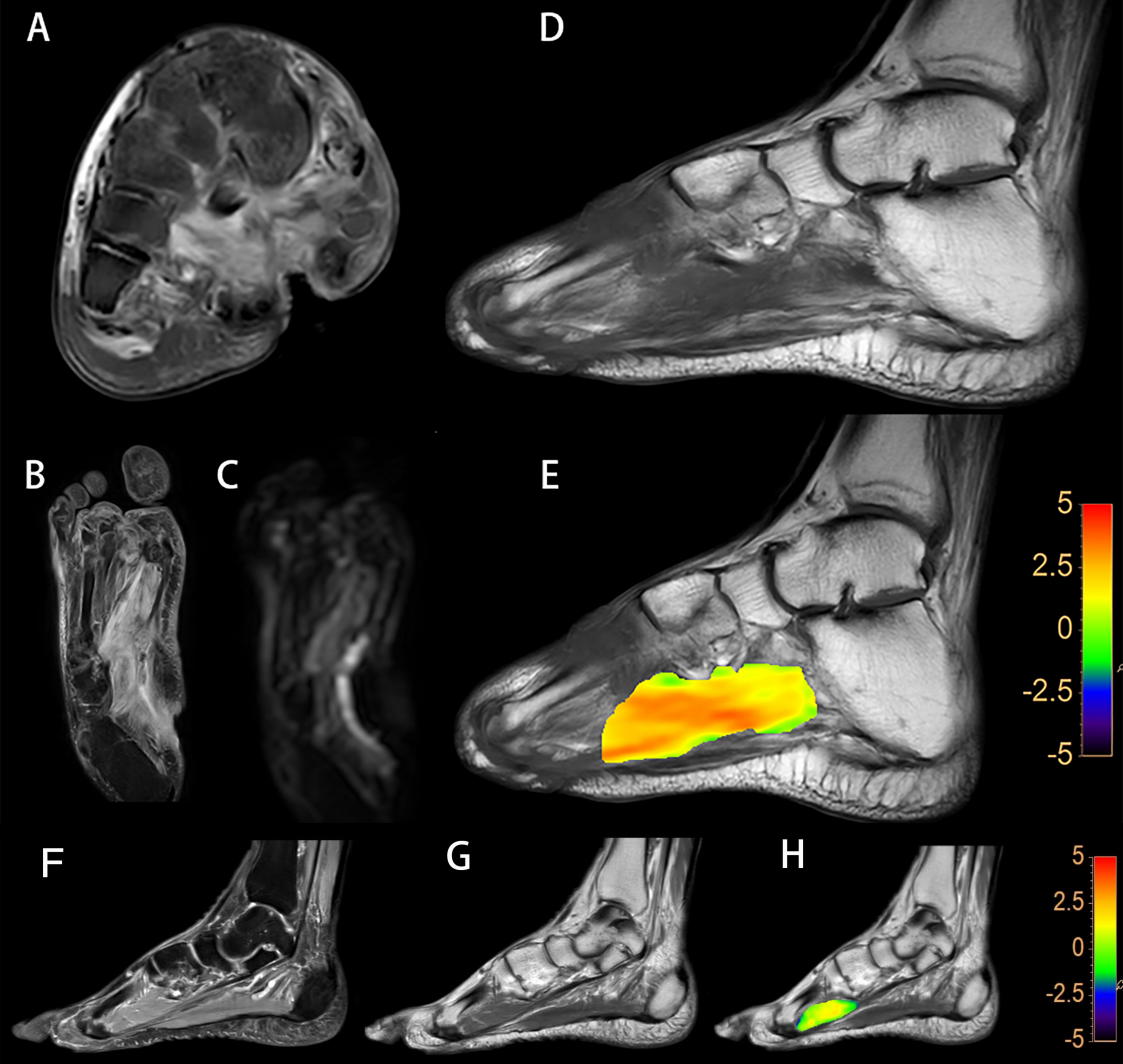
A diabetic foot of DM patient (A–E). Increased T2w signal indicates that there is soft tissue edema in the plantar and dorsum regions, and the plantar sinus tract is formed (A). Elevated proton density weighted signal indicates that there is soft tissue edema (B). Increased DWI signal in the soft tissue suggest that the water diffusion is limited (C). Lower T1w signal of metatarsal bones is considered to be osteomyelitis (D). A fused local APTw image and T1w image. MTRasym (3.5 ppm) is 3.8% (E). Images of the foot from a DM patient without DF (F–H). No abnormalities in the plantar soft tissue from the PDw image (F) and the T1w image (G). A fused local APTw image and T1w image (H). MTRasym (3.5 ppm) is 1.0%.
